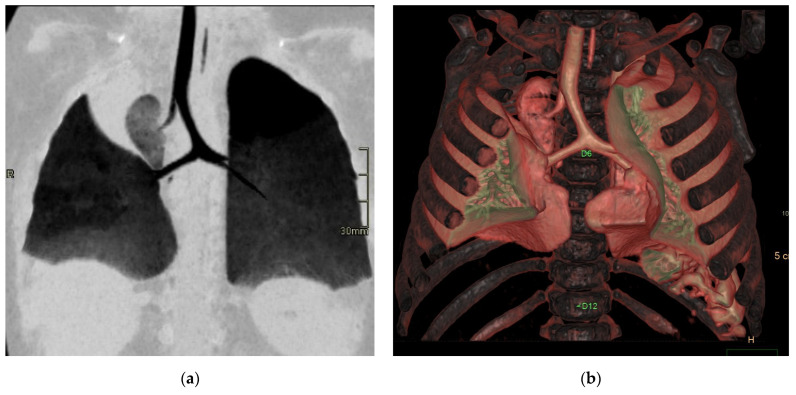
Bridging bronchus type 2. Non-contrast CT coronal (a) and VR (b) images reveal the RMB ending in a diverticulum. The right lung is ventilated by a displaced RMB arising from the LMB, forming a pseudo-carina, that is at the T6–T7 level and has an inverted T appearance.
